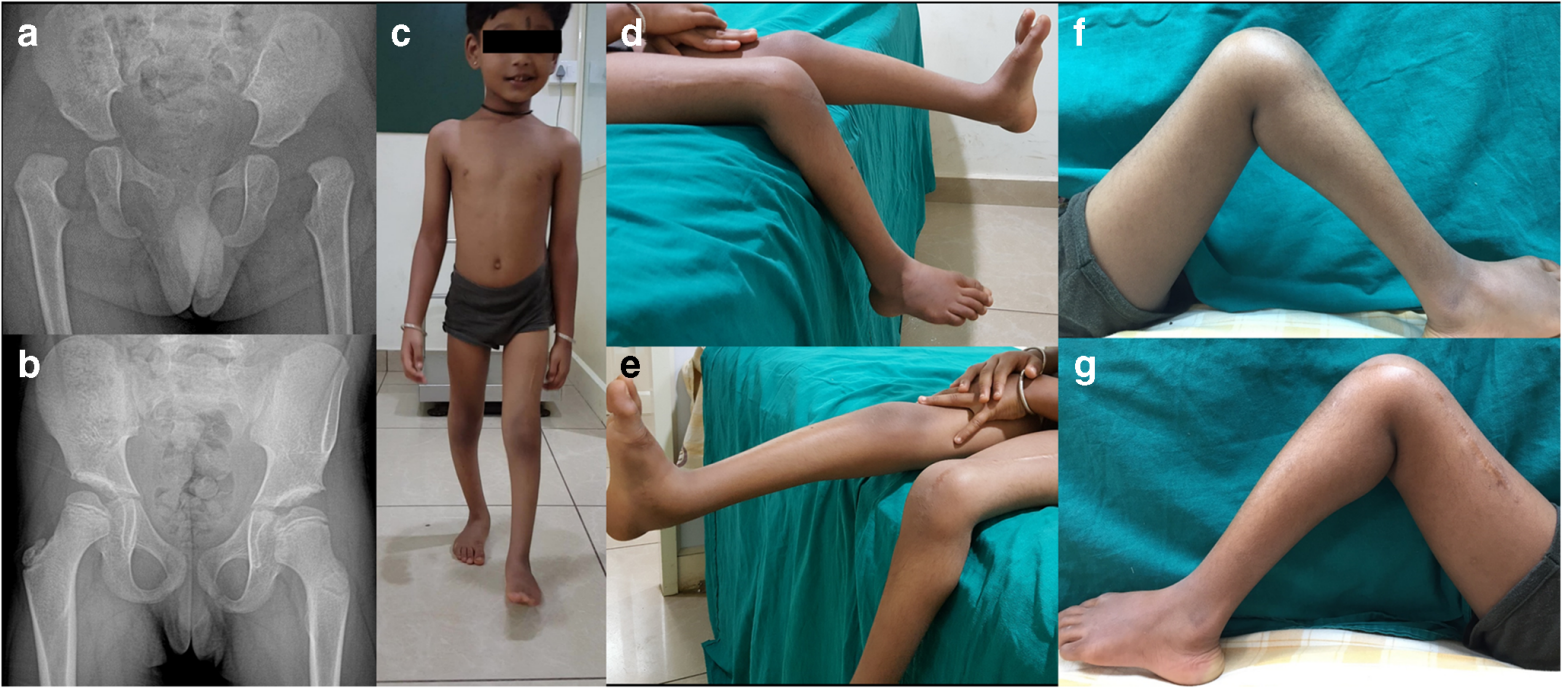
Clinical photographs and final radiographs of a child with ligamentous laxity showed good results
